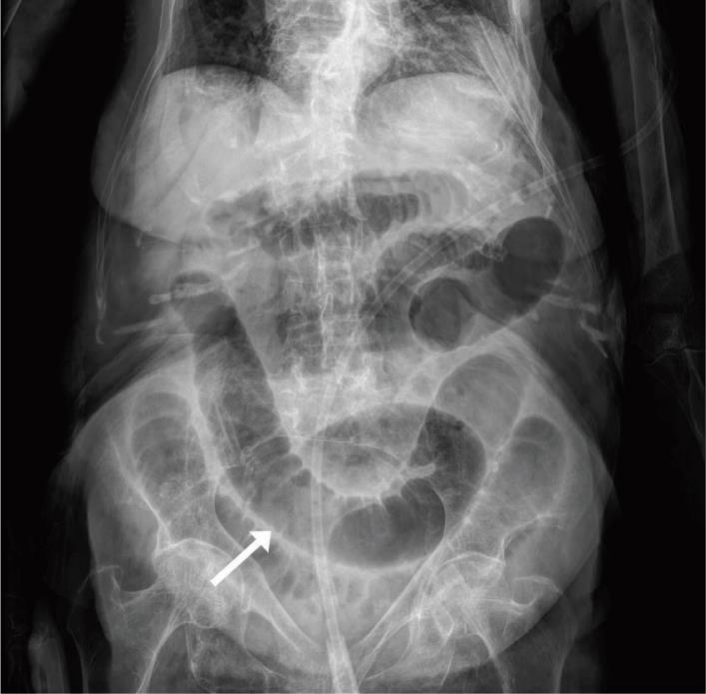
Plain abdominal X-ray demonstrating dilated loops of small bowel (white arrow), with marked valvulae conniventes and absence of gas in the rectal ampulla.
